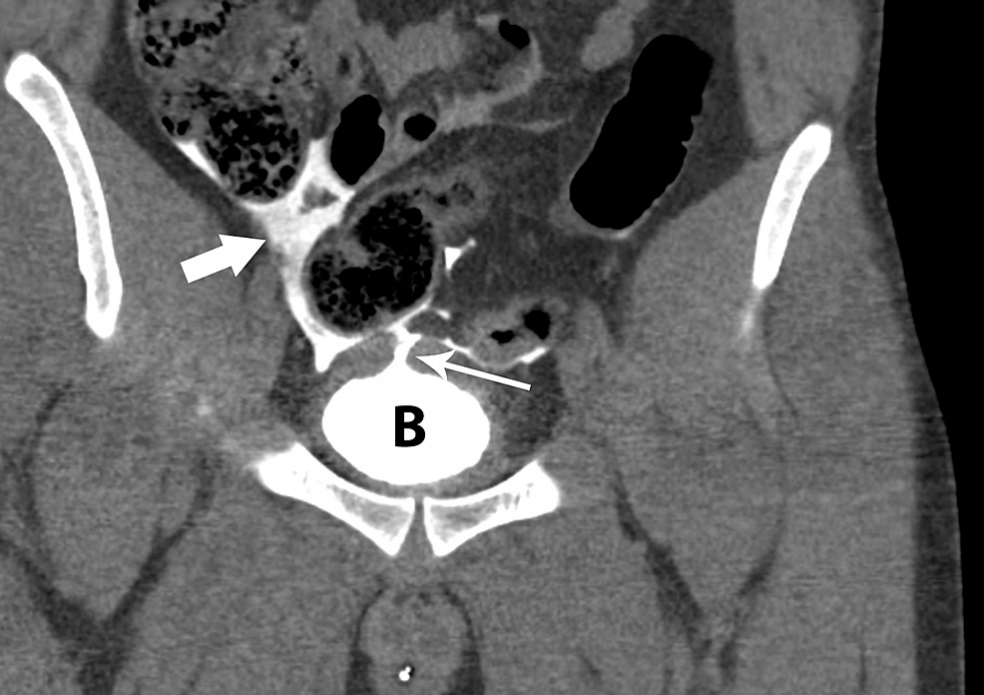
Coronal reformatted image from CT cystogram shows bladder (B) with rupture of bladder dome (thin white arrow) and extravasation of contrast material (thick white arrow) into peritoneal space.
